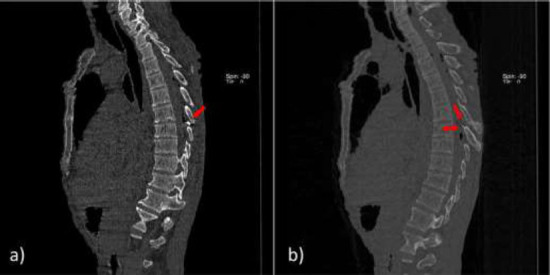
Sagittal view of thoracic CT depicting the titanium implant’s trajectory in the spinal epidural region.
